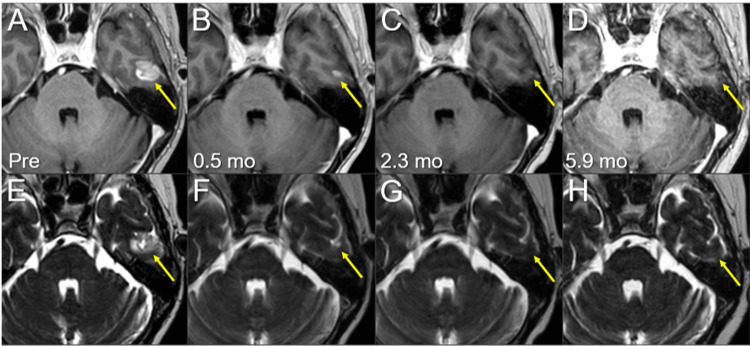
Magnetic resonance images before and after the third SRS of the left temporal new lesion. The images show (A-D) axial CE-T1-WIs and (E-H) axial T2-WIs (A, E) five days before (Pre) the third SRS (17.3 mos after the initiation of CRT); (B, F) at 0.5 mo after the initiation of the third SRS; (C, G) at 2.3 mos; and (D, H) at 5.9 mos (23.9 mos after CRT). (A-H) These images are shown at the same magnification and coordinates under co-registration and fusions. (A-H) The solid enhancing lesion (arrows in A and E) shrank remarkably at 0.5 mo (arrows in B and F) and disappeared at 2.3 mos (arrows in C and G). The lesion remained completely regressed without adverse radiation effects at 5.9 mos (arrows in D and H). CE, contrast-enhanced; WIs, weighted images; SRS, stereotactic radiosurgery; CRT, chemoradiotherapy; mo, month
